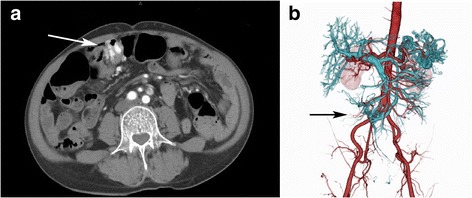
a An abdominal contrast computed tomography (CT) scan showed an artery-like vascular malformation in the wall of the sigmoid colon (arrow). b CT angiography showed an arteriovenous malformation branching from the inferior mesenteric artery and inferior mesenteric vein (arrow)
